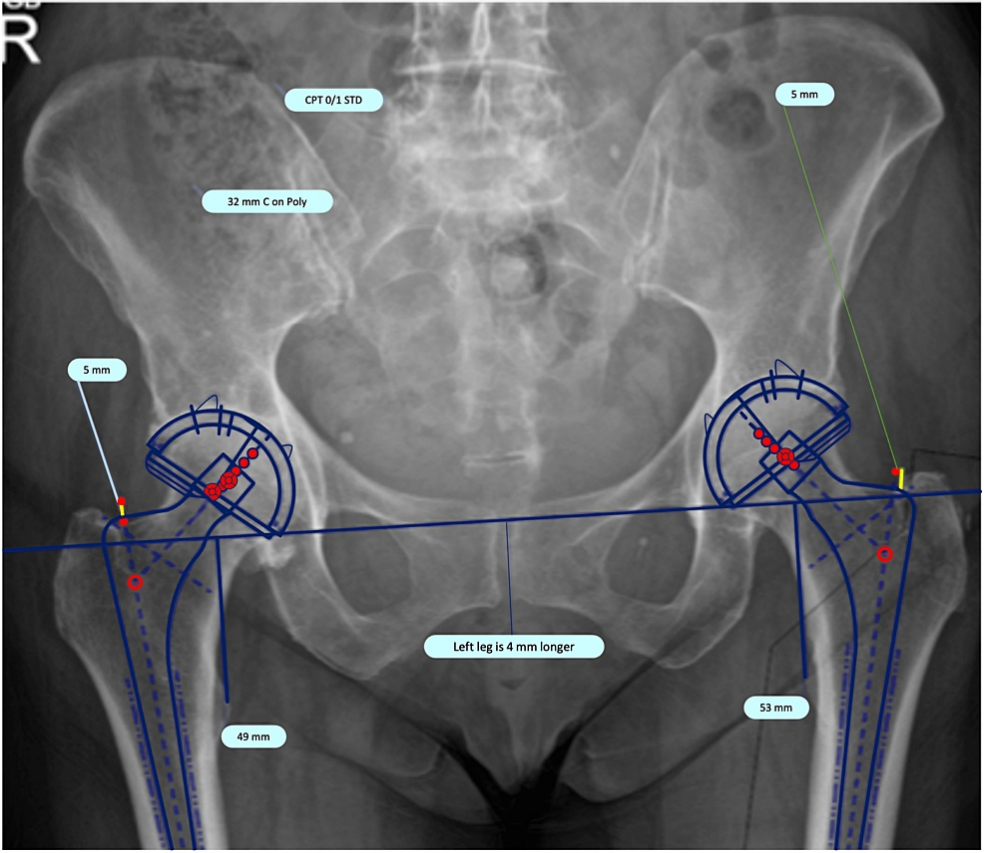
Pre-operative anteroposterior pelvic radiograph of a 45-year-old female on TraumaCad software calibrated to 118% magnification demonstrating templating technique. TraumaCad (BrainLab Inc, Westchester, IL)
